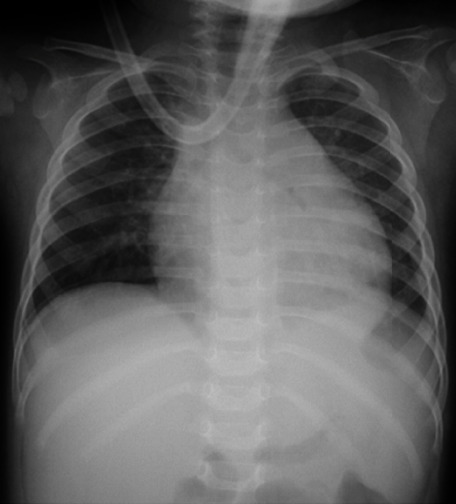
Chest X-ray obtained at admission showing an enlarged cardiac silhouette with a cardiothoracic ratio of 0.60.
